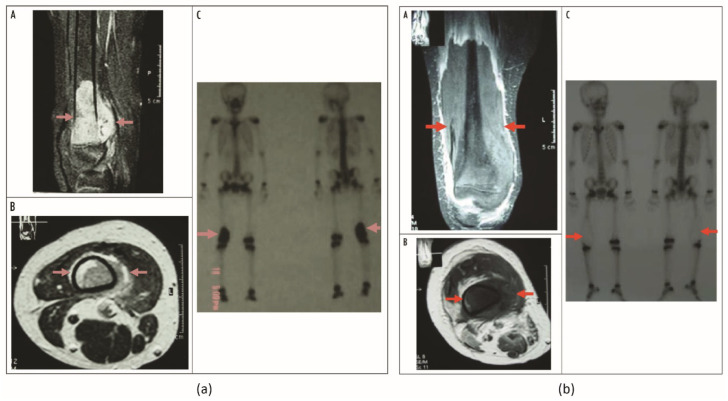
(a) Osteosarcoma in inferior segment of right femoral bone after neoadjuvant chemotherapy before HIFU. DCE-MR images obtained from (A) coronal plane and (B) transverse plane show abundant blood supply and survival tumor in the osteosarcoma lesion (red arrow). (C) Static bone imaging of ^99m^Tc-MDP, with radioactivity concentration in inferior segment of right femoral bone (red arrow); tumor survived. (b) The same patient 8 weeks after 2 HIFU treatments. DCE-MR images obtained in (A) coronal plane and (B) transverse plane show no evidence of contrast enhancement in the treated lesion (red arrow), with a papery enhanced band on the verge of inactivated area, which is indicative of complete coagulation necrosis in the osteosarcoma. (C) Static bone imaging of ^99m^Tc-MDP, with radioactivity concentration disappeared in inferior segment of right femoral bone (red arrow); tumor is inactivated. There is a reaction band along with the verge of inactivated area of tumor with higher radioactive uptake. Figures adapted from Osteosarcoma: Limb Salvaging Treatment by Ultrasonographically Guided High-intensity Focused Ultrasound, Li C, Wu P, Zhang L, Fan W, Huang J and Zhang F, Cancer Biology & Therapy, 15 June 2009, and reprinted by permission from Taylor & Francis Ltd., http://www.tandfonline.com (accessed on 7 December 2022) [41].
